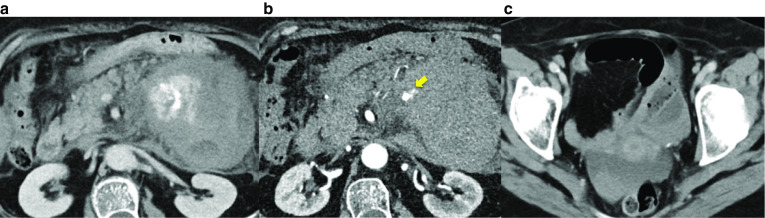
Contrast enhanced computed tomography. a The retroperitoneal hematoma and extravasation of contrast spread to the ventral side of the left kidney. b Aneurysm (yellow arrow) from a branch of the superior mesenteric artery. c Ascites in the pelvis
